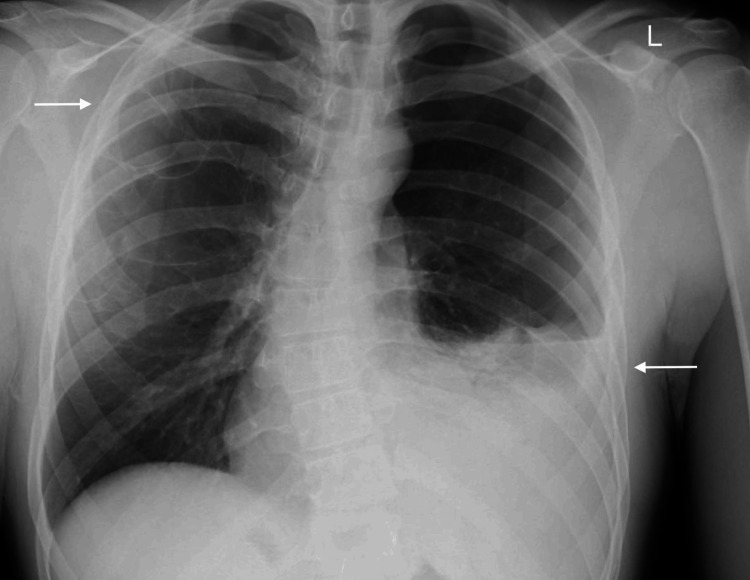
Chest X-ray showing left base effusion (lower arrow) with apical cystic bullae (upper arrow)
